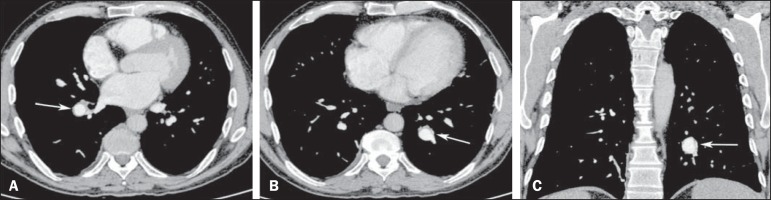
Contrast-enhanced computed tomography of the chest, with axial slices
